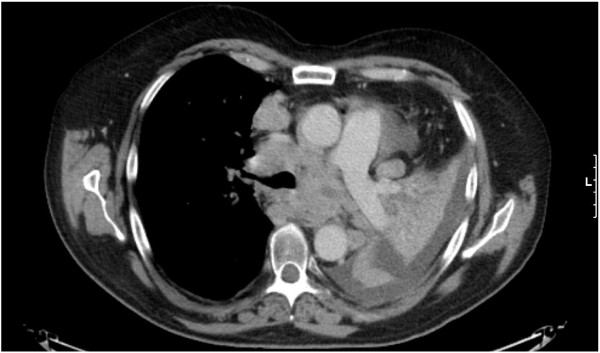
Baseline staging CT scan. CT scan of the chest prior to commencing treatment with crizotinib exhibiting extensive metastatic lung disease, mediastinal lymphadenopathy and a partially collapsed left lung.
